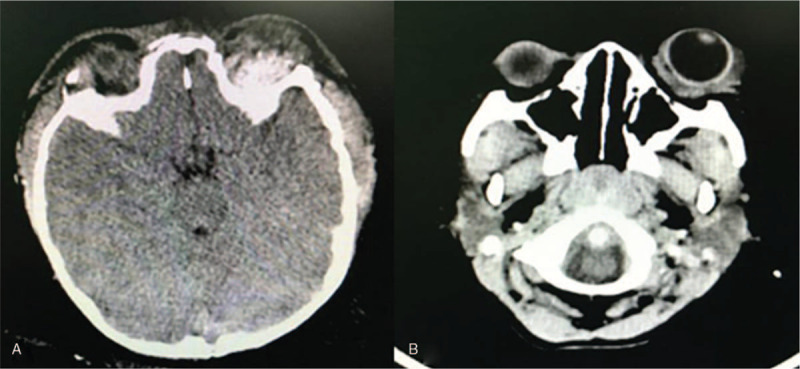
Cranial computed tomography scan after fronto-orbital advancement. (A) Retrobulbar epidural hematoma. (B) Left proptosis.
